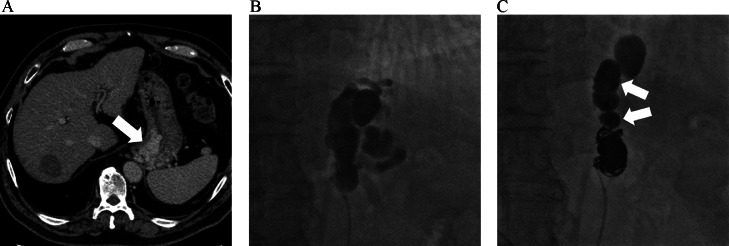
A 72-year-old patient with gastric varices (case 1). (A) Contrast enhanced computed tomography before *n*-butyl cyanoacrylate-assisted retrograde transvenous obliteration showed gastric varices protruding into the stomach (arrow). (B) After inserting a balloon catheter into the gastrorenal shunt, 5% ethanolamine oleate iopamidol was injected under balloon occlusion. (C) Because the insertion of 11 long coils did not completely embolize the gastrorenal shunt, *n*-butyl cyanoacrylate (arrow) was injected through a microcatheter under balloon occlusion.
